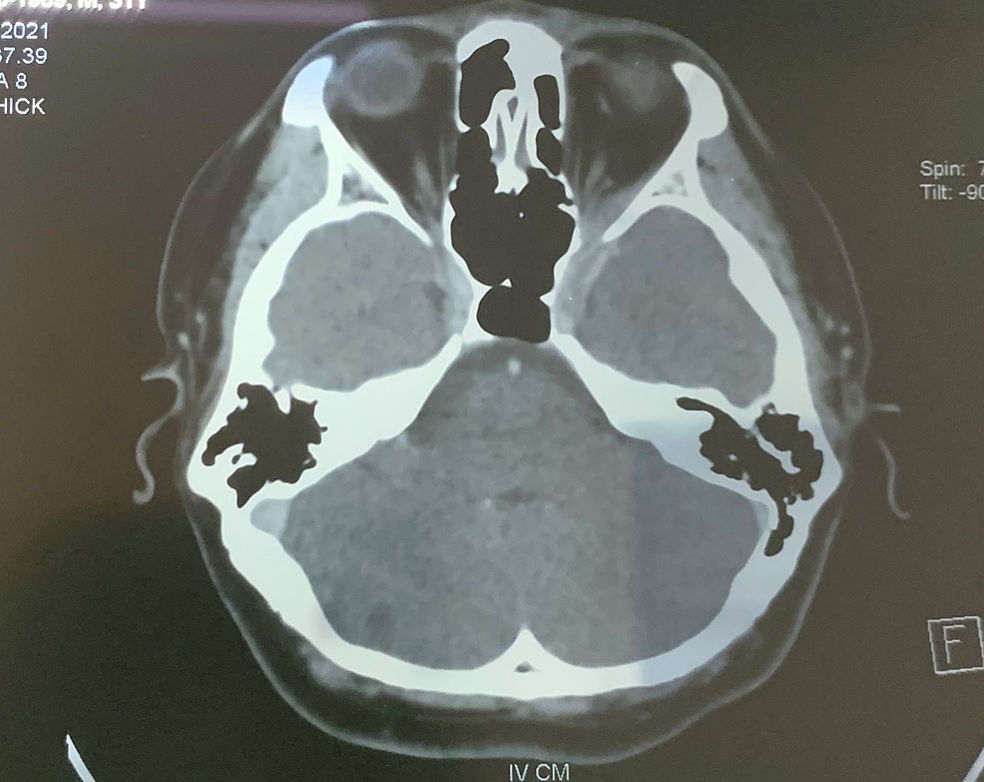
An axial CT scan view showing left cavernous sinus diffuse thickening with filling defect and enhancement extending anteriorly to the left orbital apex.
